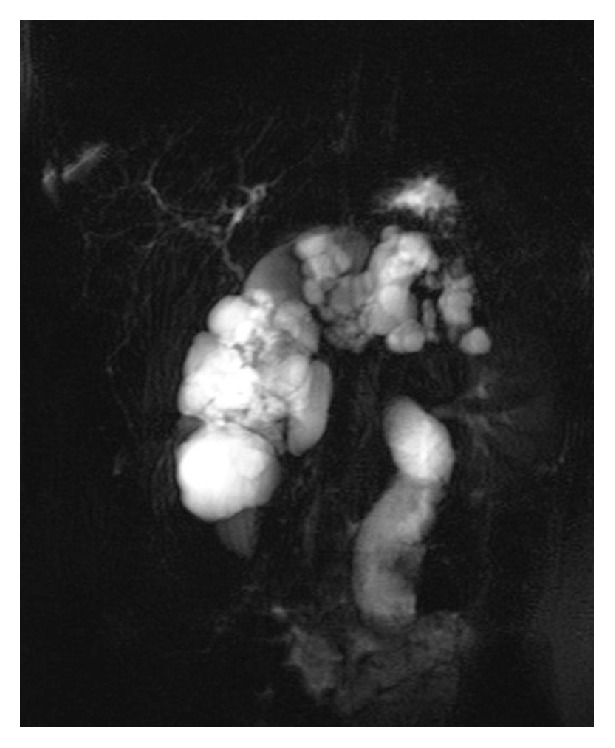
MRCP. T2-weighted MR image—HASTE (Half-Fourier Acquisition Single-Shot Turbo Spin Echo) sequence—demonstrates complete replacement of the pancreas by multiple cysts of variable size with thin and smooth walls. There is no communication of cysts with pancreatic ducts.
